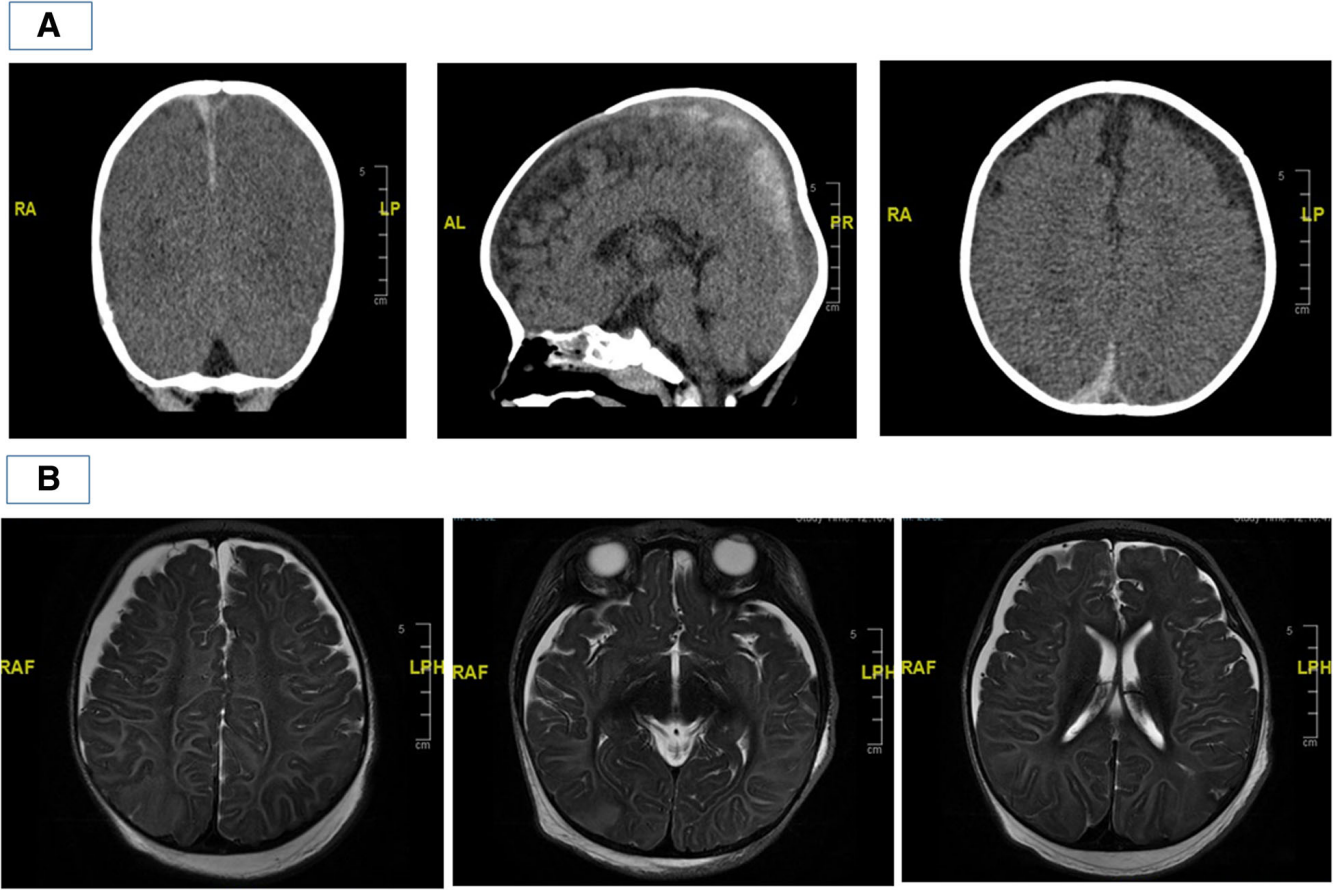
a CT brain done at Children’s Emergency showing bilateral subdural haemorrhages. b MRI brain done two days off ECMO (T2-weighted images) showing patchy areas of cortical swelling and reduced grey-white differentiation in the respective lobes; leftmost image: right parietal lobe; middle image: occipital lobes; rightmost image: left parietal lobe
